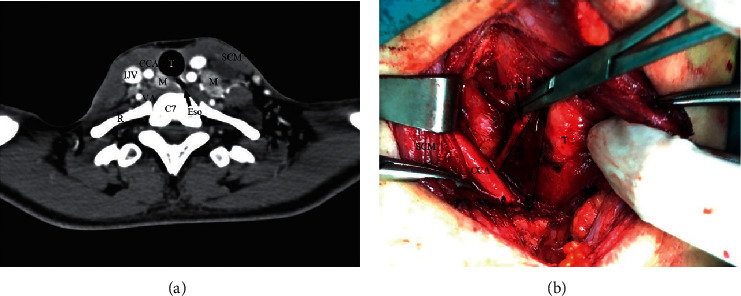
Axial contrast-enhanced CT of LN-prRLN in the cervical space. Axial contrast-enhanced CT scan shows that the LN-prRLN (M) is medial-posterior to the level of the CCA and IJV and anterior to the prevertebral fascia (aside the tracheoesophageal sulcus). B. The surgical field after LN-prRLN dissection during right-sided central compartment dissection. The part within the quadrangle with solid lines presents the detailed anatomic structure of the LN-prRLNlevel. The right CCA, IJV, and SCM were retracted laterally with a long-arm retractor. CCA: common carotid artery; IJV: interjugular vein; SCM: sternocleidomastoid muscle; T: trachea; VA: vertebral artery; C7: 7^th^ cervical vertebra; R: first rib; Eso: esophagus.
